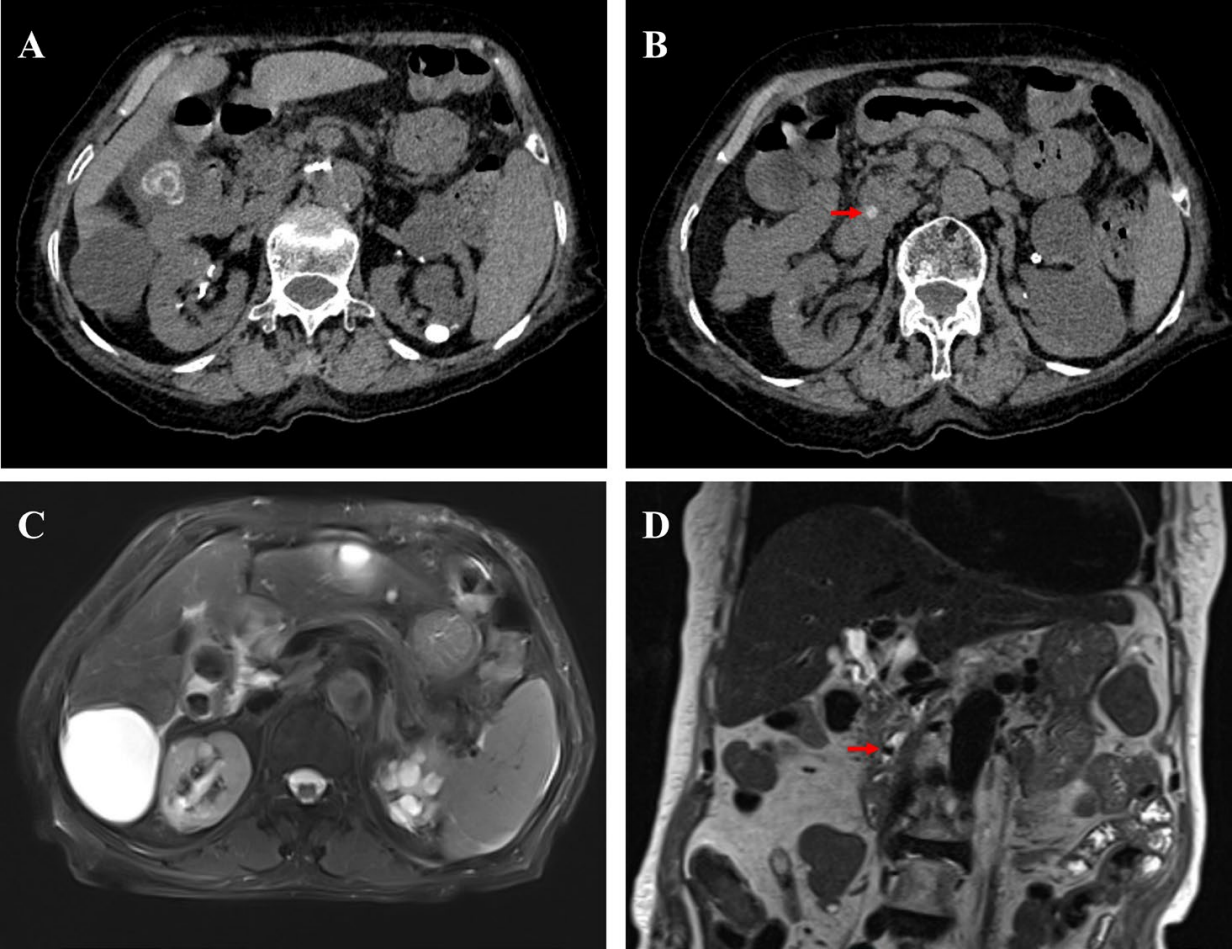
CT and MRCP features before treatment of the patient: CT scan showing gallstones and hepatic cysts (A) and a calculus in the distal common bile duct (B); MRCP scan showing gallstones and hepatic cysts (C) and a calculus in the distal common bile duct (D). Red arrow indicates the calculus in the distal common bile duct.
